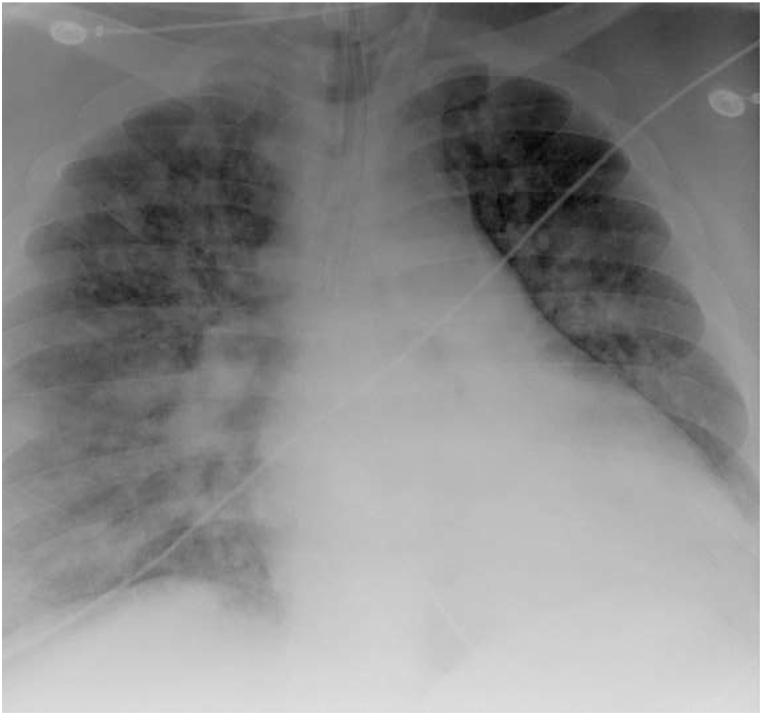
Post EM Titration. Endotracheal tube tip is about 3.9 cm above the carina. NG tube overlies the mediastinum, the tip not seen, and can be confirmed by abdominal radiograph. Diffuse bilateral patchy airspace disease, similar to prior. No large effusions or pneumothorax. Unchanged cardiac size.
